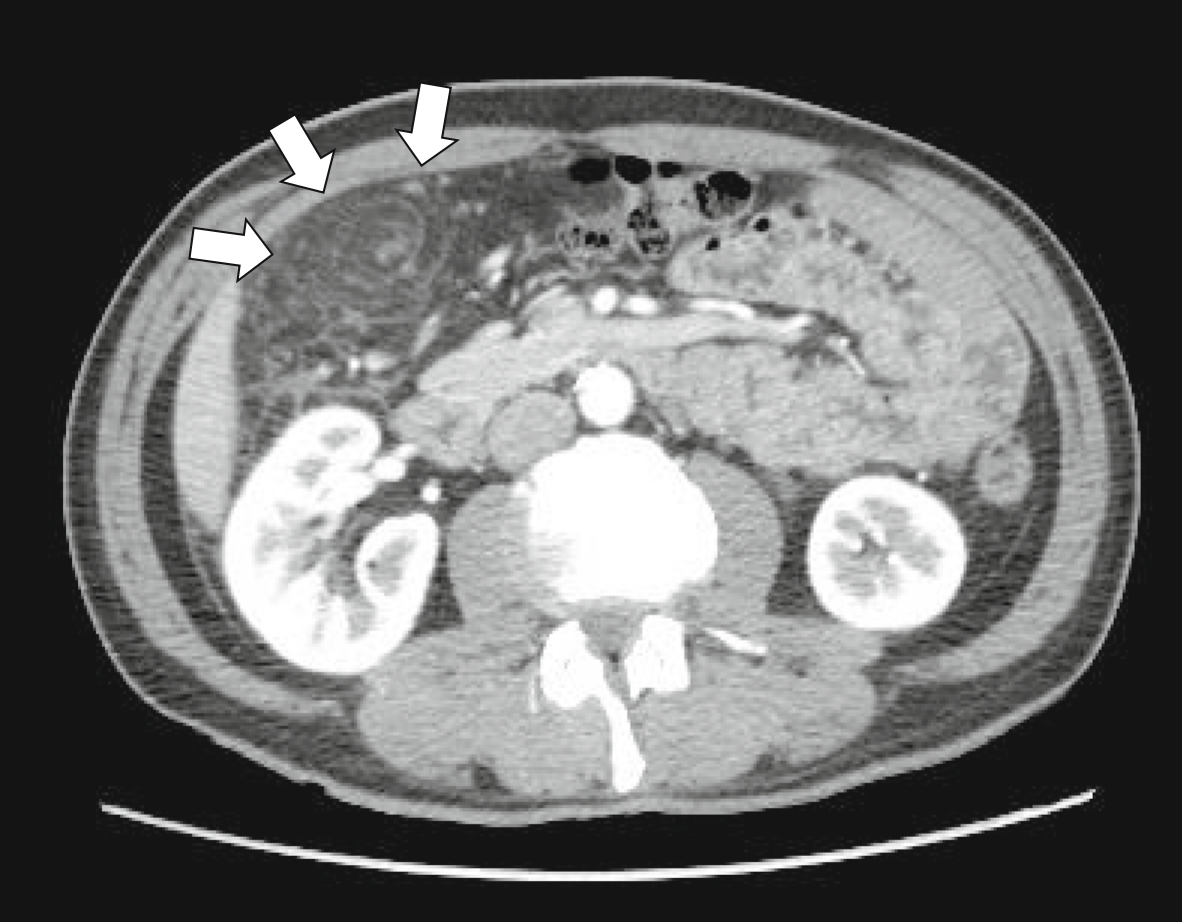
Preoperative abdominal computed tomography scan. Twisting of the omentum with a local mass of fat density and fluid in a whirling oval-shaped mass pattern at the right flank and iliac fossa (white arrow) was observed. The fatty mass is situated between the transverse colon and the gallbladder and contains hyperattenuating streaks
